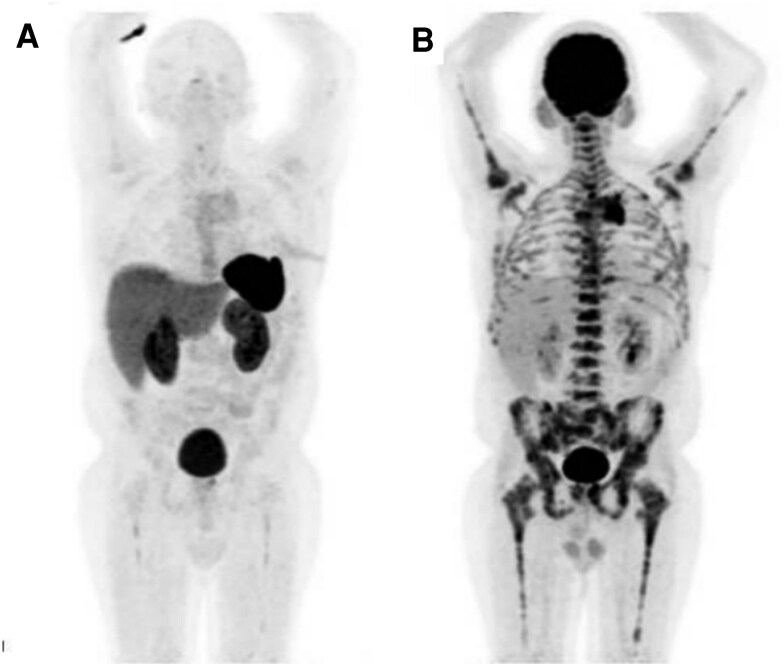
(A) 18F-NOTA-octreotide PET/CT with low uptake in the remaining tissue. (B) 18FDG PET/CT with increased uptake in the mediastinal lesion, mediastinal lymph nodes and bone lesions.
